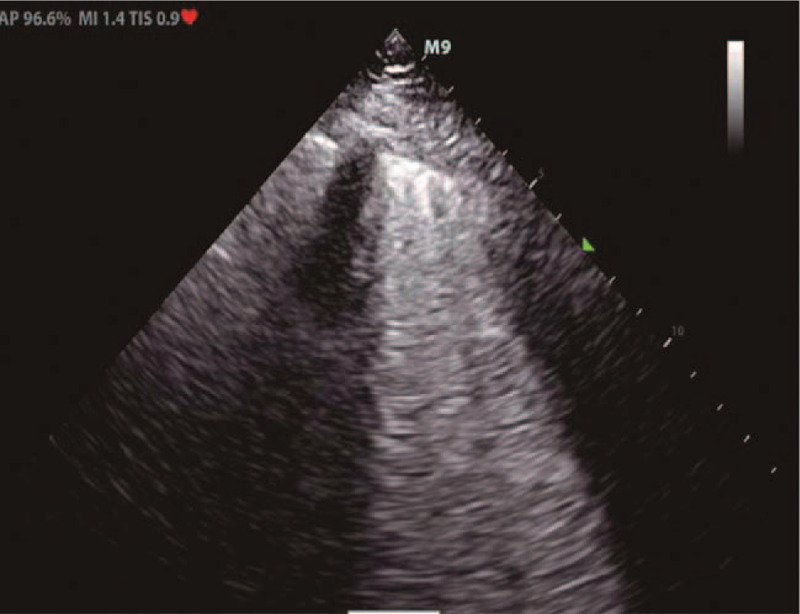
Lung ultrasonography showing a large number of B lines.
